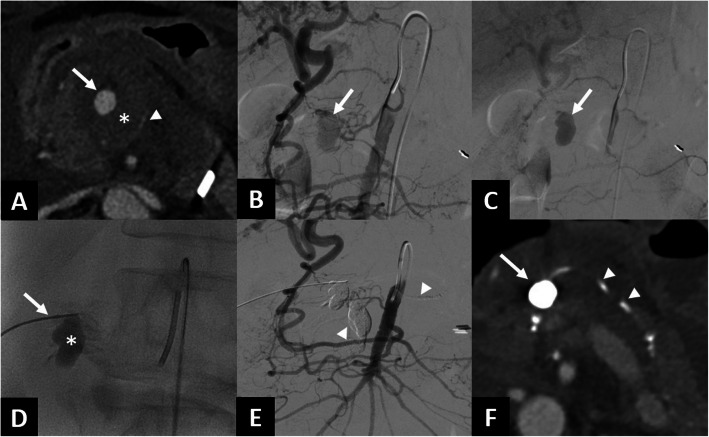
79-year-old male referred to the emergency room for abdominal pain (Patient n°4). A The abdominal CT scan in the axial section at arterial phase revealed a retro-pancreatic hematoma (*) resulting in compression of superior mesenteric vein (arrowhead) and a saccular aneurysm of the dorsal pancreatic artery (arrow). B Angiography of the SMA using a Sidewinder catheter confirmed the saccular aneurysm and demonstrated retrograde vascularization of the liver. C The aneurysm showed persistent late opacification (arrow). D After the technical failure of TAE, the aneurysmal sac (*) was punctured under ultrasound guidance using a 22G needle (arrow). E After percutaneous embolization using a Glubran®/Lipiodol® mixture, opacification of the SMA no longer showed opacification of the aneurysm and revealed non-target embolization (arrowheads). F The follow-up CT scan one week later confirmed satisfactory occlusion with Lipiodol® deposition, despite non-target embolization along the DPA. Abbreviations: CT, Computed tomography; DPA, Dorsalpancreatic artery; SMA, Superior mesenteric artery; TAE, Transarterial embolization
